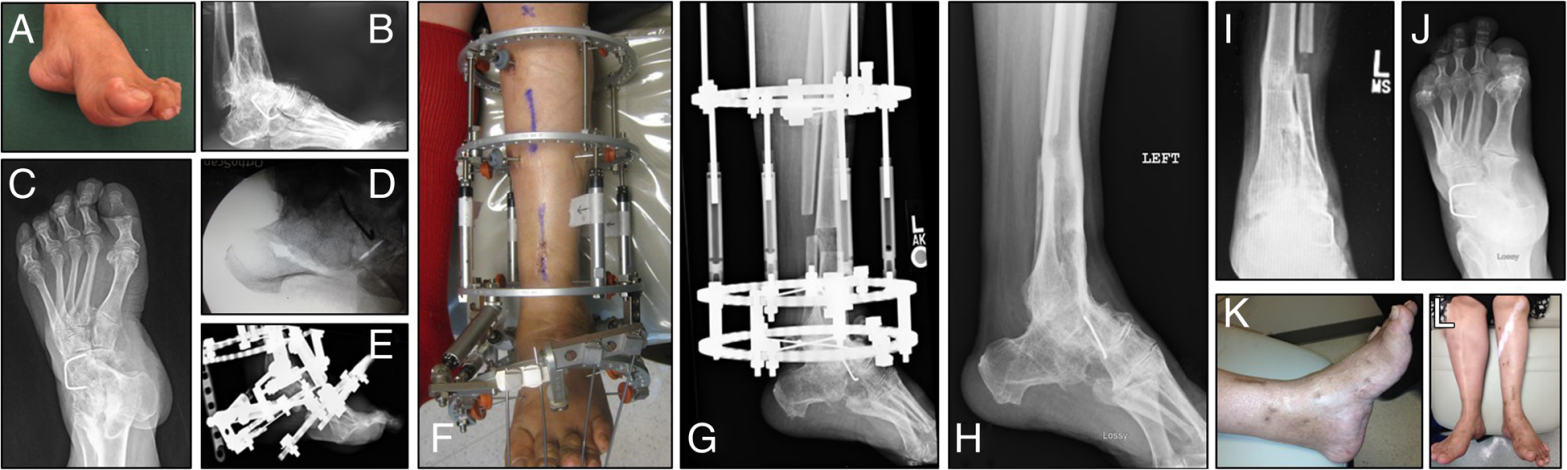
Case #7. Fifty-eight-year-old F with history of clubfoot correction as a teenager. a Preoperative appearance of foot. b, c Preoperative radiographs of cavoadductovarus foot with evidence of prior surgery. d Intraoperative fluoroscopic image of V osteotomy. e–g Clinical and radiographic appearance of frame construct for gradual foot correction and DTC demonstrating regenerate formation. h–j Postoperative anteroposterior and lateral radiographs demonstrating foot correction and consolidated distal tibial corticotomy site. k, l Postoperative appearance of the foot and corrected LLD
